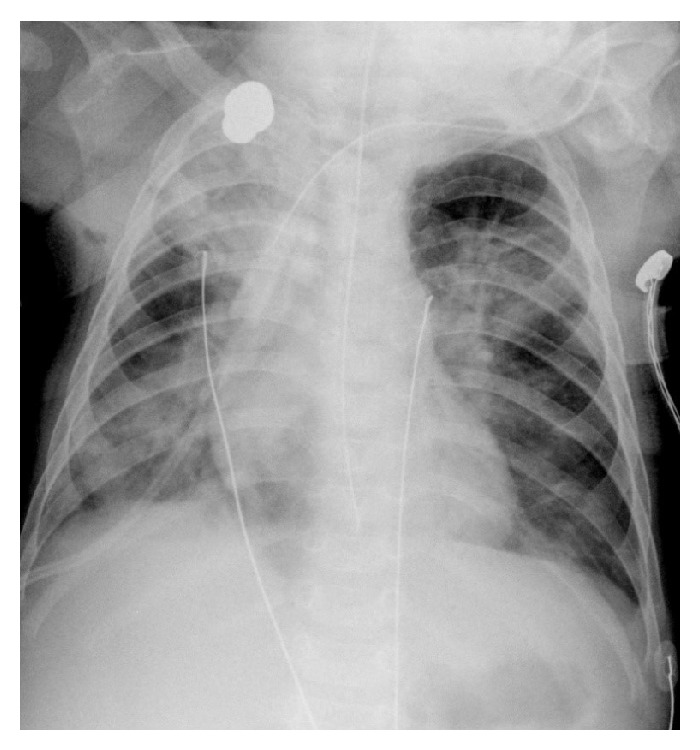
Thoracic X-ray on admission.
